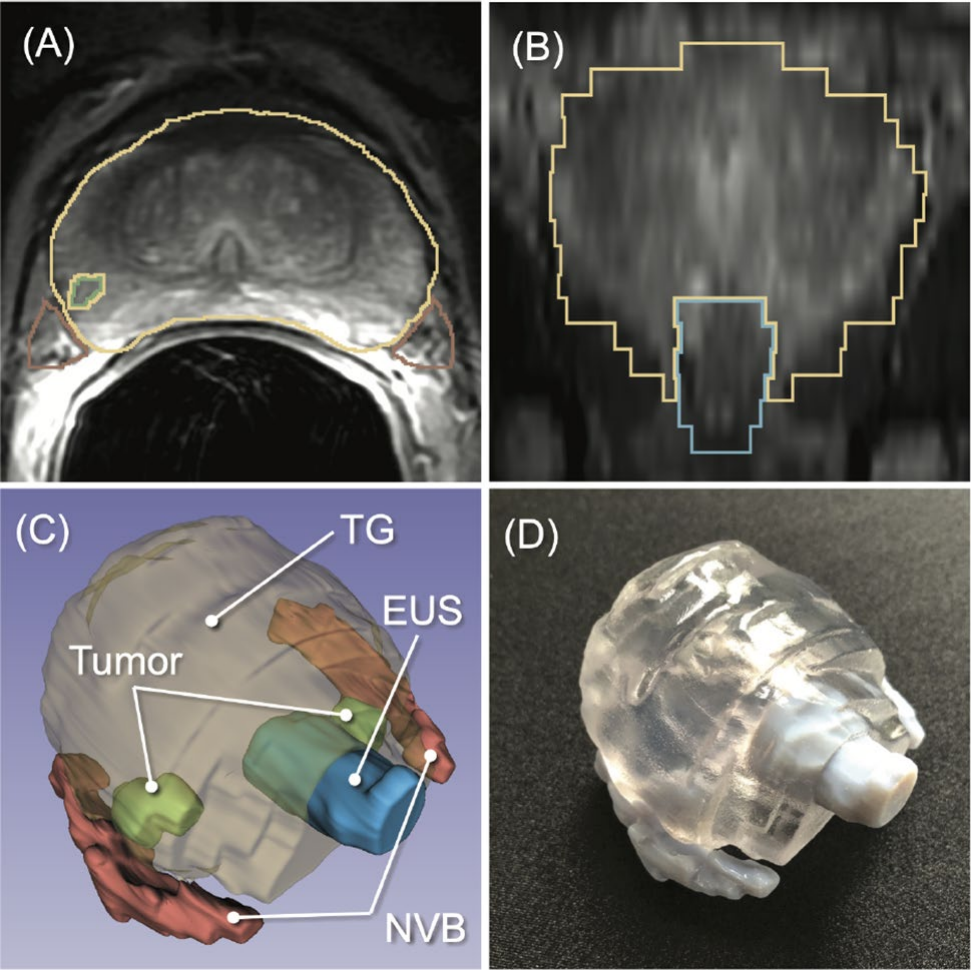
An example application of 3D segmentation of the prostate and adjacent structures for surgical planning. The prostate gland, neurovascular bundle (NVB), external urethral sphincter (EUS), and tumor are manually segmented on the preoperative T2-weighted MRI (A, B) by a radiologist, and then converted to a 3D surface model (C). The model can also be 3D-printed (D) for surgical planning, and preoperative communication with the patient.
